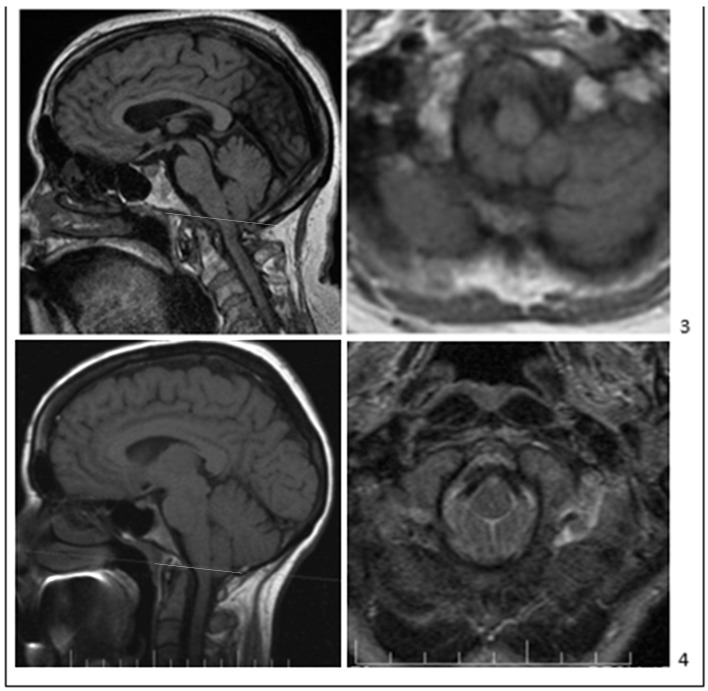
Types of CM0 without syringomyelia. The McRae line (white line) is shown on the T1-weighted sagittal images (left panels). Axial images at McRae’s line are shown (right panels) on T1-weighted (3) and T2-weighted (1,2,4) images. Adult symptomatic CM0-only patients with tonsillar herniation ≤2 mm (panels (1–3)) and borderline CM1-only patients with tonsillar herniation 3 mm (4). All patients demonstrate short bones (CL and SO < 40 mm), crowdedness of the PCF, tight foramen magnum, and CM1-like clinical manifestations: transient localized suboccipital cough-related headaches (1) or constant and transient suboccipital headaches (2–4), truncal ataxia, vertigo, dizziness, and upper motor neuron signs. The sagittal images in (1,3) (left panels) also show spinal canal narrowing at the level of the odontoid.
